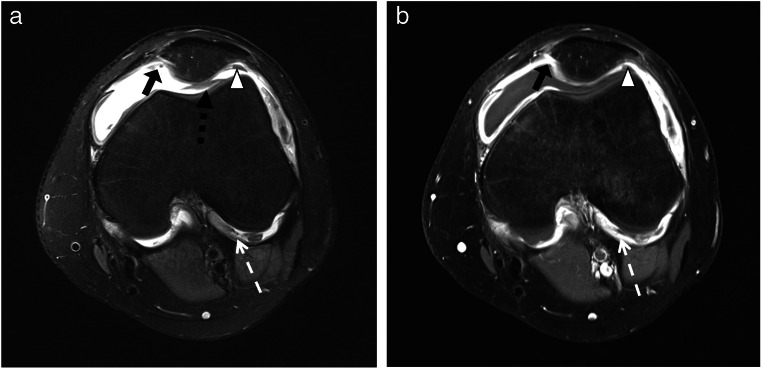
Twelve-year-old girl with extended oligoarthritis. Axial images demonstrating joint effusion and synovial membrane thickening, notably at the junction of the lateral condyle and the cruciate ligament (white dotted arrow), medial (black arrow), lateral (white arrowhead), and dorsal (black dotted arrow) to the patella, notably visible on image. a Proton-density-weighted fat-saturated sequence. b Contrast-enhanced T1-weighted turbo spin echo fat-saturated sequence
